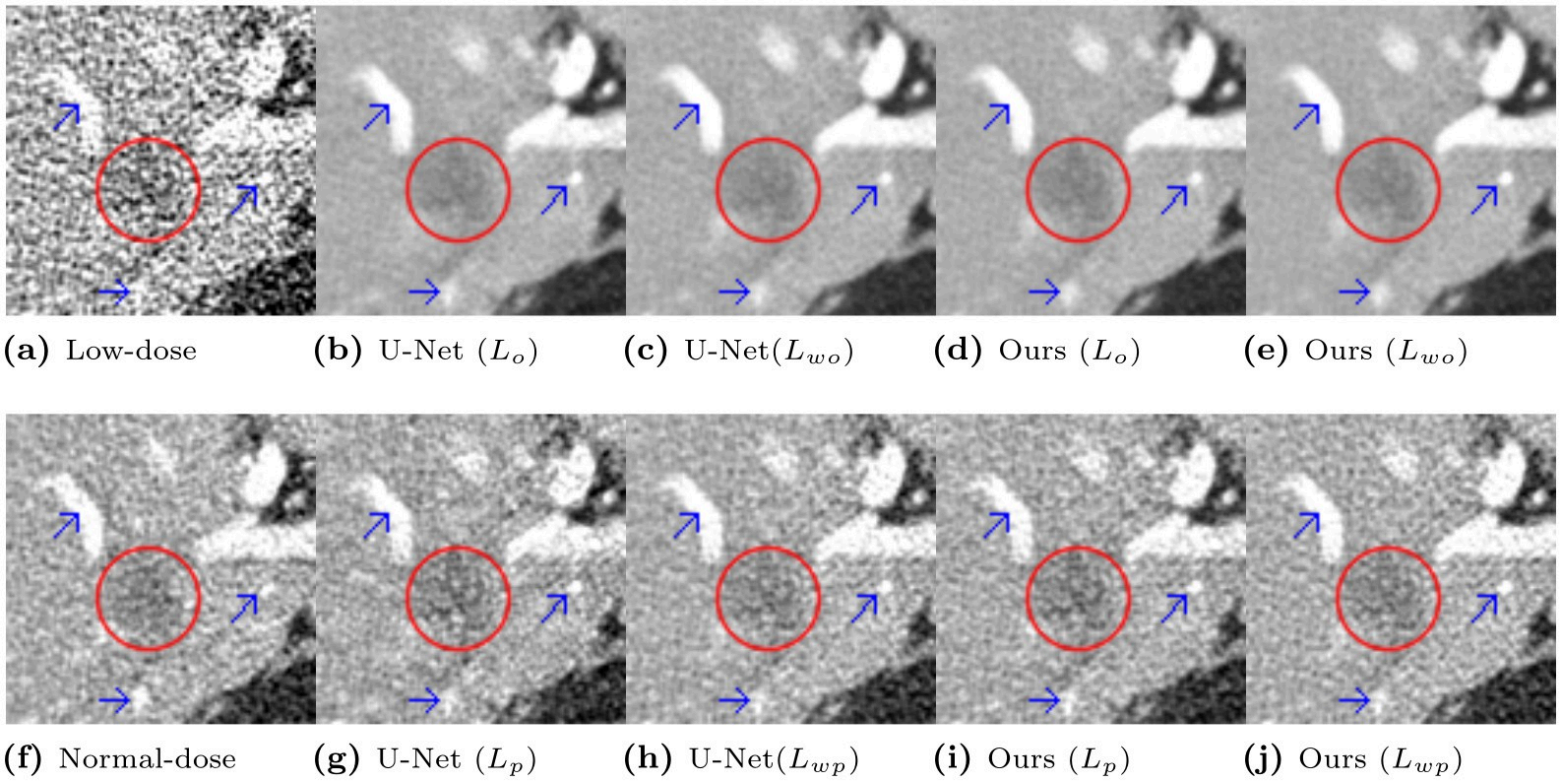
Denoised CT images from U-Net and the proposed methods with various loss functions. Images in (b), (c), (d), and (e) are from the networks that optimized the objective loss functions. Images in (g), (h), (i), and (j) are from the networks that optimized the perceptual loss functions. The display window is [-160, 240]HU. Red circles and blue arrows point to the low attenuation lesion and blood vessels in the posterior right liver lobe, respectively.
